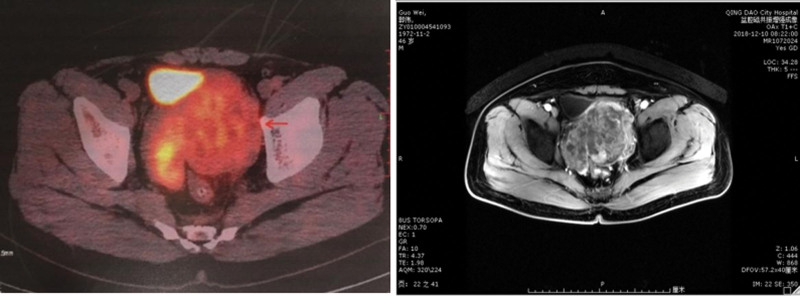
PET/CT and MRI of the primary tumor. CT = computed tomography, MRI = magnetic resonance imaging, PET = positron emission tomography.
